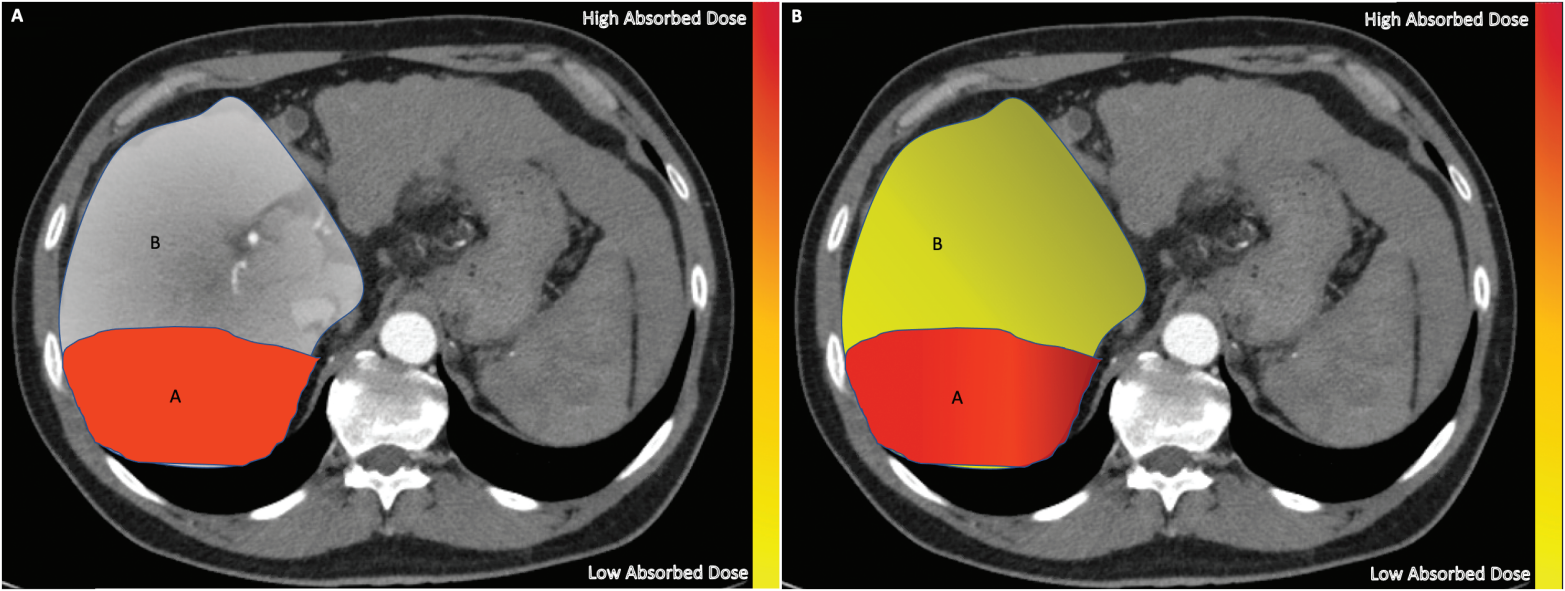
(2A): Contrast-enhanced CT demonstrates highlighted right hepatic lobe which contains two compartments A and B. In this radiation segmentectomy, all of the Y90 is delivered to the perfused segments VI and VII which can be modeled by the MIRD equation. No absorbed dose is seen in the non-perfused segments V and VIII (B). Absorbed dose scale is provided on left for reference with red indicating highest dose and yellow indicating lowest dose. (B) Contrast-enhanced CT demonstrates highlighted right hepatic lobe which contains two compartments A and B. In this radiation lobectomy, the Y90 is delivered to the two compartments per the partition equation. The tumor takes up a higher absorbed dose as expected. The nontumorous liver takes up a lower dosage as expected. Absorbed dose scale is provided on left for reference with red indicating highest dose and yellow indicating lowest dose.
